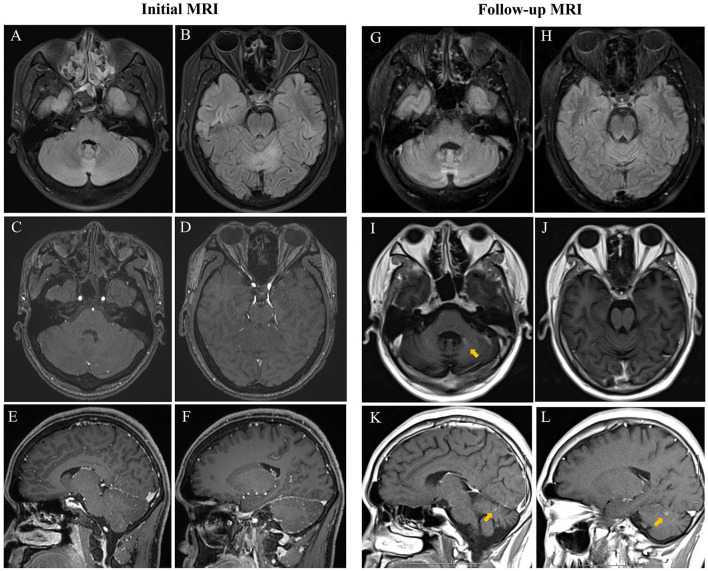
Initial cerebral MRI showed increased signal in the vermis and both bilateral cerebellar hemispheres on FLAIR (A,B) without enhancement on contrast-enhanced T1-weighted sequence (C–F). Repeated MRI showed an increase of FLAIR hyperintensity of cerebellar lesions (G) and progression in size of the vermis lesions (H) with slightly enhanced T1-weighted signals on both axial and sagittal view and obvious cerebellar atrophy (I–L).
